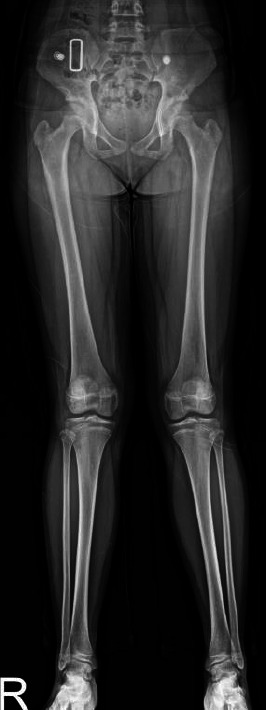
Whole extremity AP radiograph of a 12-year-old female. Whole extremity AP radiograph shows bilateral genu valgum and ankle valgus deformity.
